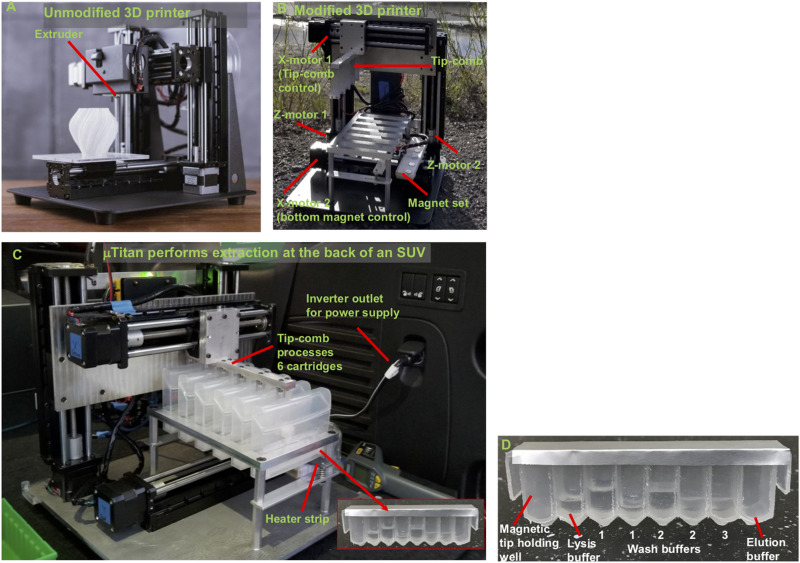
Concept of converting a low-cost 3D printer to perform rapid and automated NA isolation. (A) A typical FMD type 3D printer. (B) A 3D printer turned extraction device. The extruder of a 3D printer was removed to allow the adaptors to be mounted. The magnetic particle processing tip-comb (tip-comb) was attached on the mount. Its vertical and lateral movements are controlled by two Z-motors and one upper X-motor controls. Another X-motor control below the extraction cartridges enable resuspension of magnetic particles for NA binding and washing (see description later). The tip-comb has 6 fingers with each finger has magnets to use magnetic coupling to externally control the extraction tips inside an extraction cartridge to perform extraction. There is no direct contact between the magnets, the samples, and MPs. Six samples can be processed simultaneously under the same protocol program. The heated strip under the elution wells uses the 3D printer’s extruder heater and thermistor to provide precise temperature control for heated NA elution. (C) μTitan placed inside the cargo area of an SUV. (D) A reagent strip that has been pre-filled with the appropriate extraction reagents.
